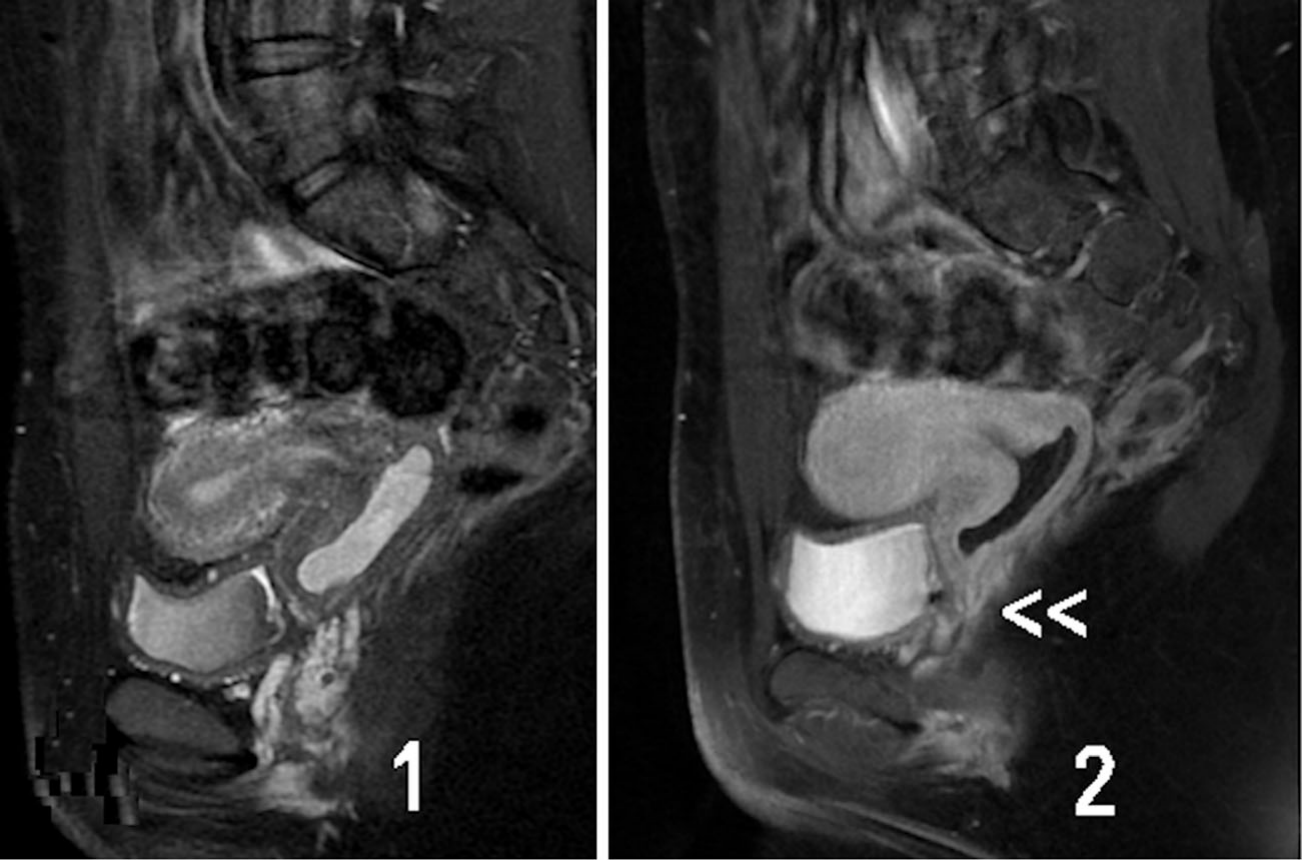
Urogenital sinus anomalies. MR images [(1) T1- and (2) T2-weighted fat-supressed MR in the sagittal plane] showing a retrovesical blind vagina with apparent inferior half atresia and undetected fistula tract to the bladder (<<) in a 28-year-old patient. Cystoscopy confirmed the presence of an orifice situated in the trigone, just above the bladder neck, equidistant and below both ureteral meati, through which menstrual blood clearly exited from the vagina. The patient suffered from cyclic menuria and the opening of the fistulous tract into the bladder trigone was in fact the hymen (courtesy of Dr. JC. Martínez-Escoriza, Alicante)A *rectovestibular fistula* often coexists with vaginal or vestibular atresia. Female cloacal exstrophy occurs when the urorectal septum fails to separate from the cloacal membrane, resulting in the urethra, vagina, rectum and anus opening into a single common channel (Fig. 8b). MR could accurately demonstrate the level of bowel termination in patients with persistent cloaca in addition to its high sensitivity for detection of Müllerian anomalies, which are present in 73 % of patients [68].6.*Malformative combinations*: Some patients may present several associated anomalies of mesonephric, Müllerian and/or cloacal origin [9, 54, 69] that result in very complex malformations [70, 71] with a rich chart of symptoms that may be difficult to appropriately catalogue and treat, especially if the embryology and physiopathology of the female genital tract is not taken into consideration.
